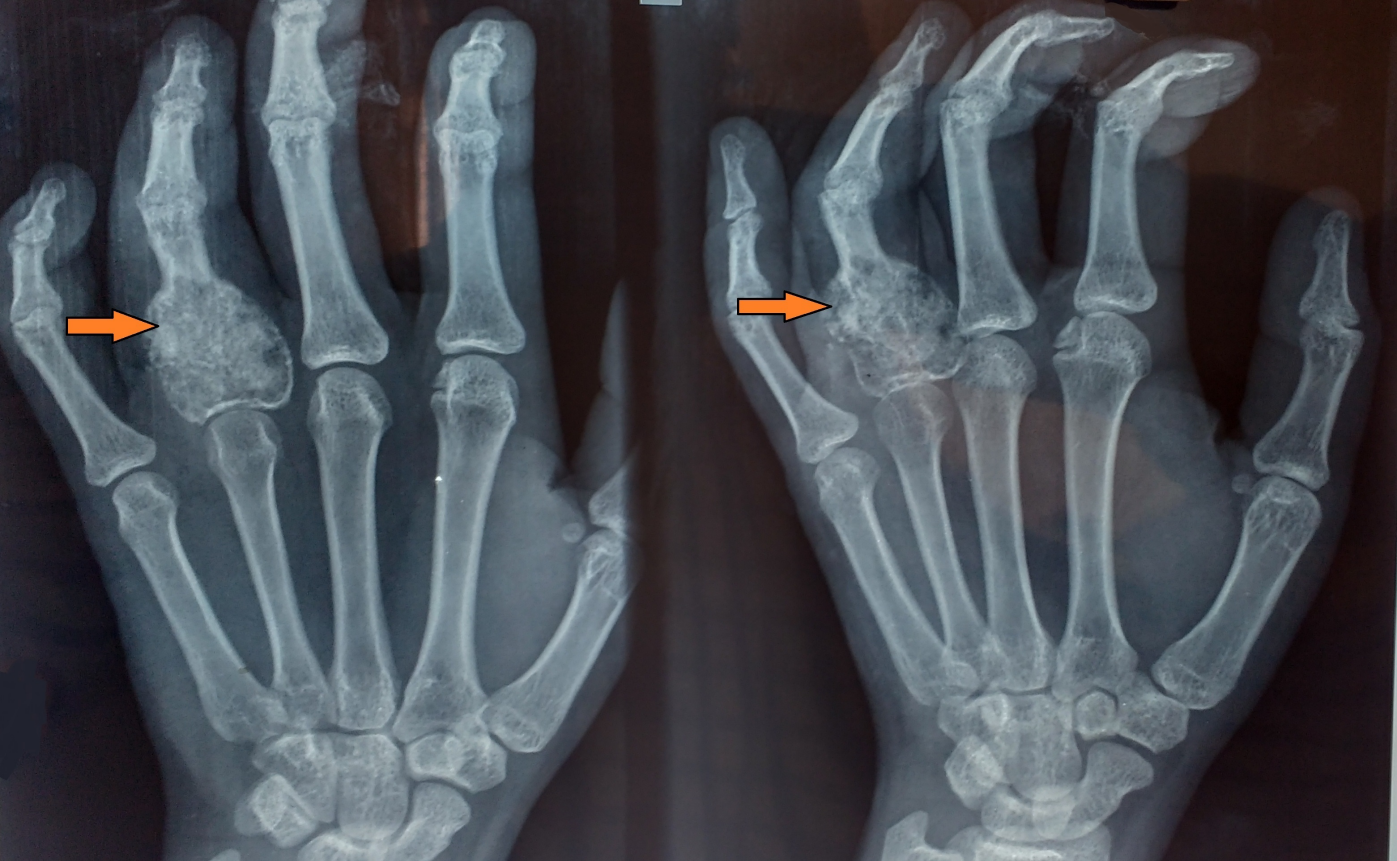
Post-op radiograph Shows curettage of the lesion and the bone grafting done
